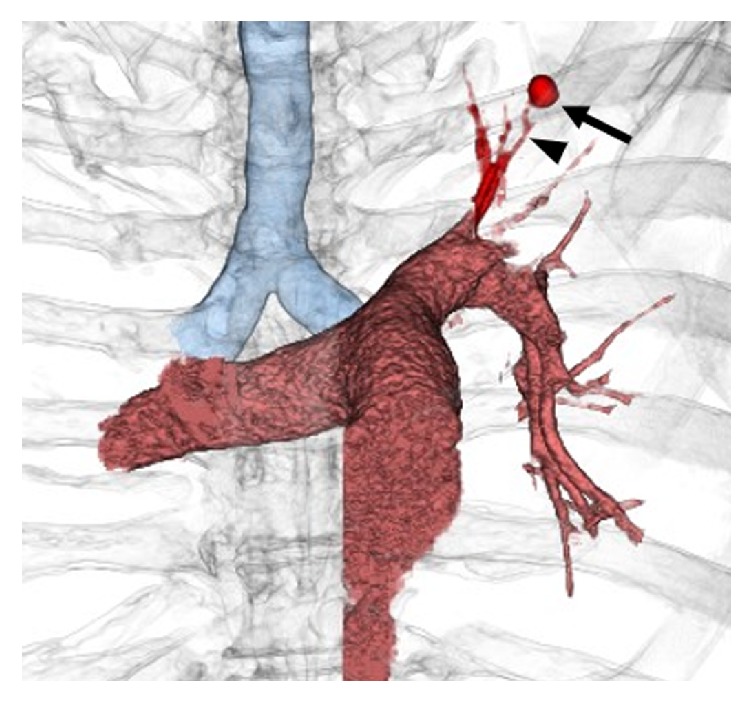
Volume rendering reconstructed image of the computed tomography angiography. The aneurysm (arrow) seems to originate from a branch of the left superior segmental pulmonary artery (arrowhead).
